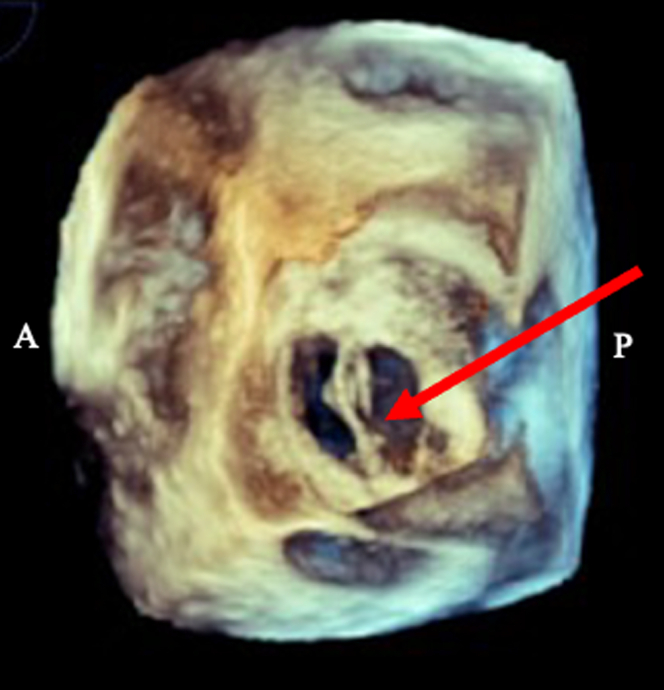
Three-Dimensional Transesophageal Echocardiography of the Fenestrated Atrial Septal Defect Three-dimensional image of the fenestrated atrial septal defect (as seen from the left atrium) demonstrating adjacent septal fenestrations and the intervening tendon ridge (red arrow). A = anterior; P = posterior.
